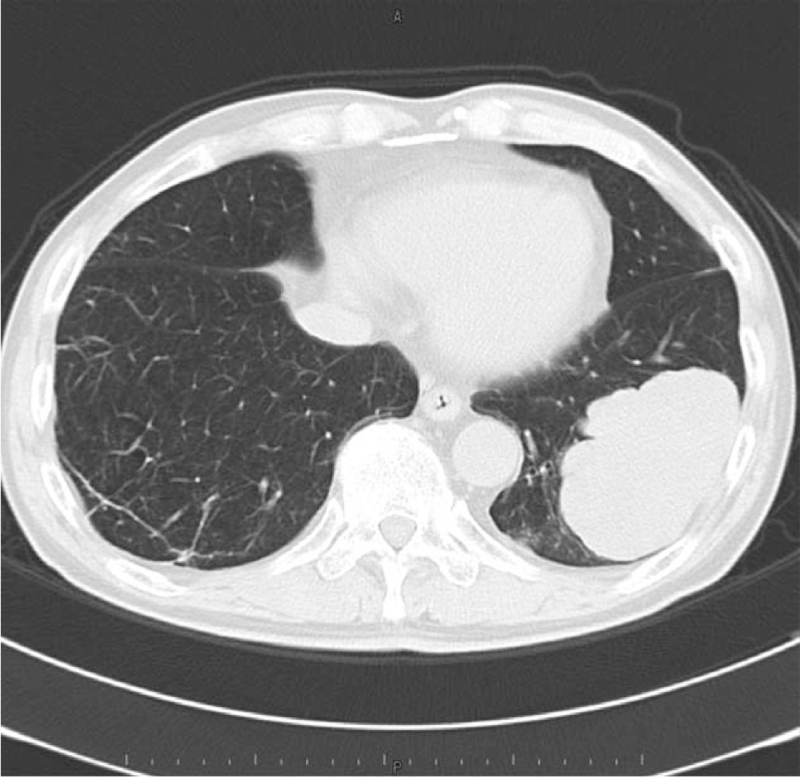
Axial chest computed tomography (CT) (lung window setting) showing 8.5 cm mass shadow in the left lower lobe, and many flat nodules along with the left pleura.
